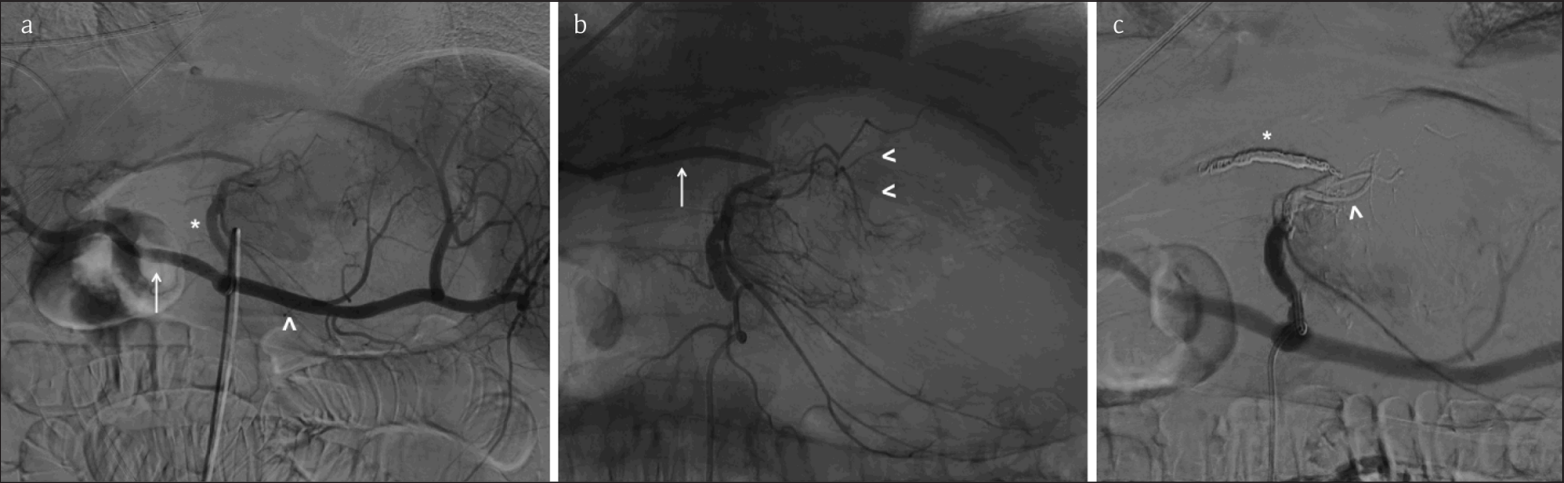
Selective arteriography of the celiac trunk (CT) in a patient addressed for massive upper bleeding from a gastric tumor. (a) Asterisk: left gastric artery (LGA); arrow: common hepatic artery (CHA); arrowhead: splenic artery (SA). (b) Superselective catheterization of the LGA showing a common anatomical variant with a left hepatic artery (LHA, arrow) originating from the LGA. On the left size, the branches supplying the lesser curvature of the stomach (arrowheads). (c) Embolization of the LGA is performed in this case in two steps: firstly, embolization of the LHA by coils (asterisk) to protect the liver; then, injection of a mixed oil Lipiodol-glue to obtain distal occlusion of the gastric bleeding (arrowheads). The LGA is the target in bariatric embolization for the treatment of the obesity; three ongoing trials are evaluating the outcomes of this new treatment modality, using coils and particles (see Table 1).
